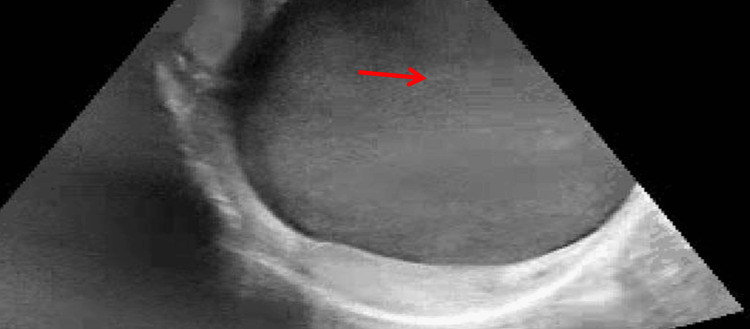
Scrotal ultrasound demonstrating left-sided hematocele Ultrasound of the left scrotum demonstrating an enlarged, complex fluid collection (arrow), consistent with a hematocele
